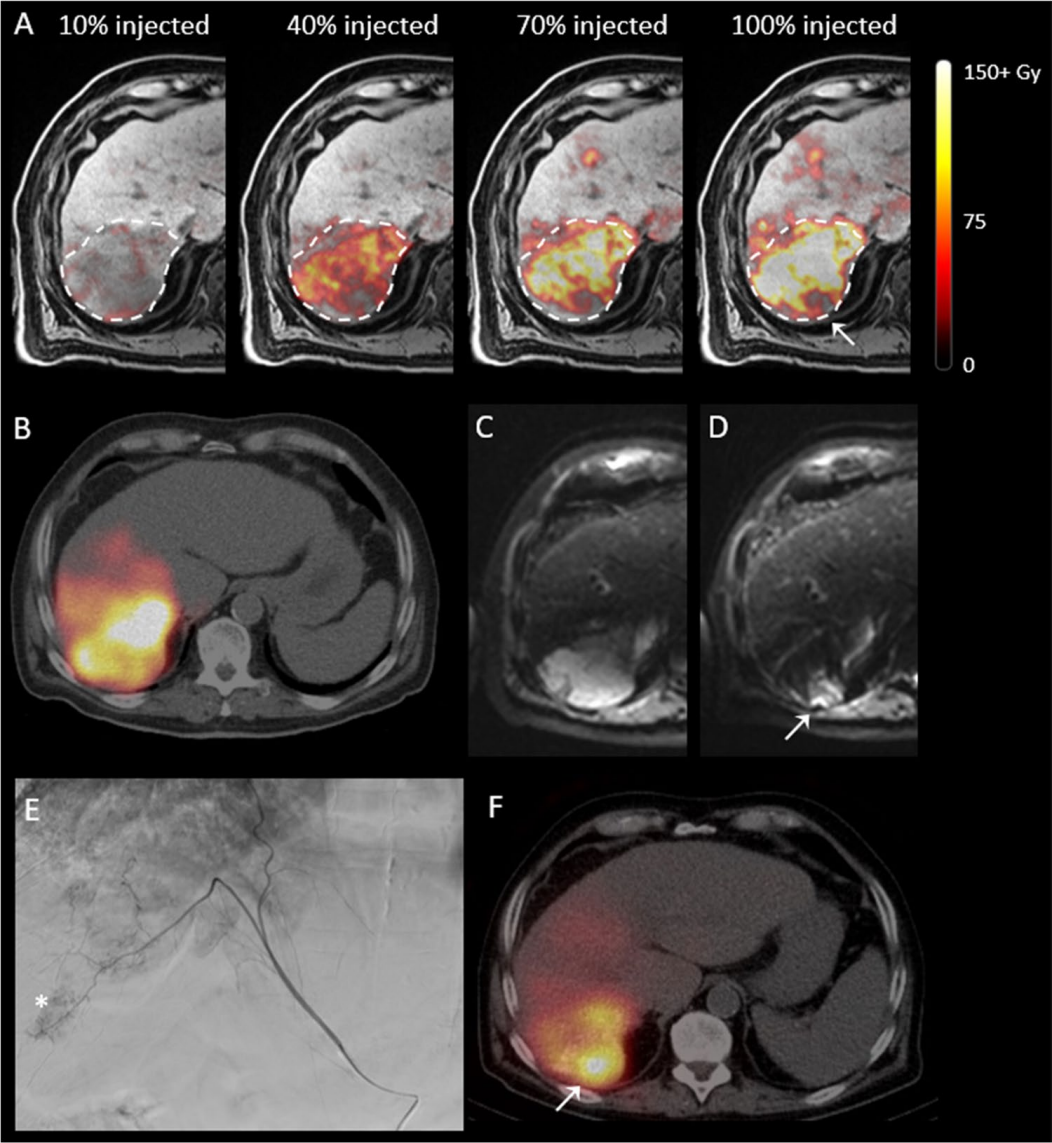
A tumour (hepatocellular carcinoma) of patient 4 treated via the right hepatic artery, in which a small part that was vascularized through an aberrant vessel originating from the phrenic artery remained untreated. A MRI-based dose distributions after each of the four fractions of radioactive microspheres had been administered. The tumour is delineated with the dashed line, and the arrow indicates the untreated area. B
